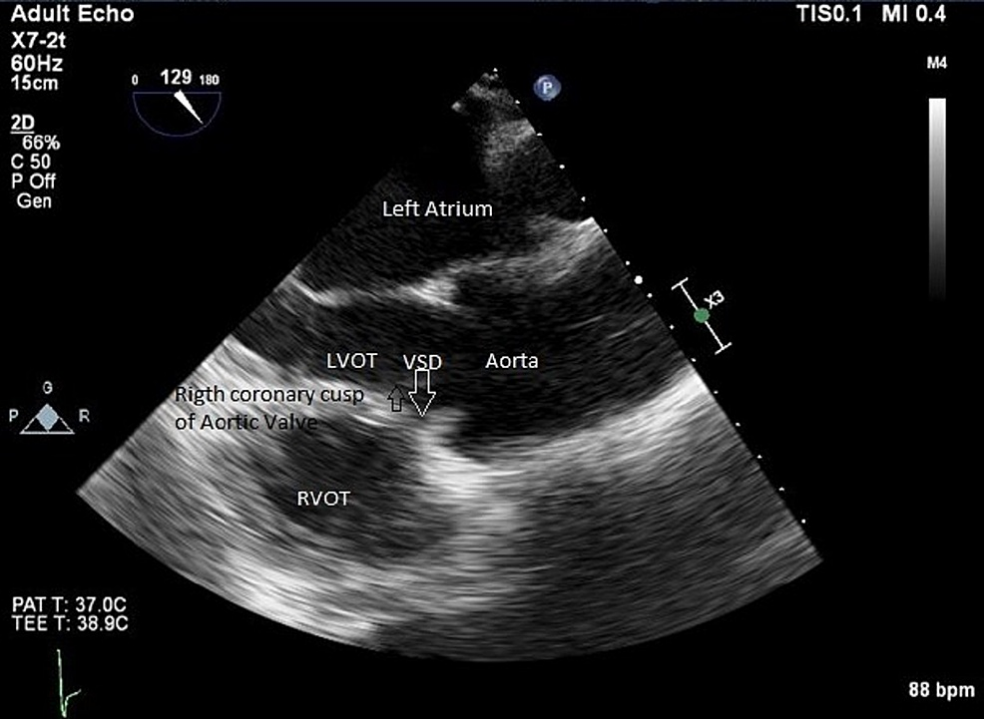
Transesophageal echocardiogram long-axis view of the aortic valve without color flow showing the left to right shunt through the outlet VSD and right coronary cusp prolapse VSD: ventricular septal defect; LVOT: left ventricular outflow tract; RVOT: right ventricular outflow tract The white arrow denotes VSD and the black arrow denotes the right coronary cusp of the aortic valve.
